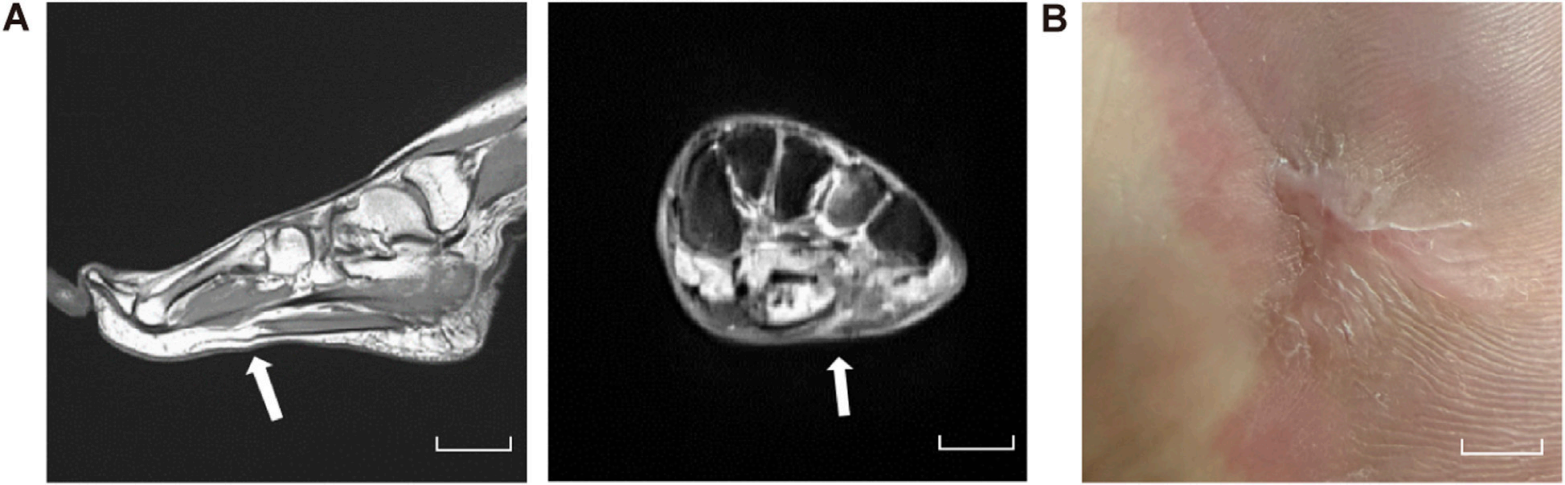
At the 1-year follow-up. (A) MRI of the left plantar foot. Scale bars, 5 cm. (B) Latest figure of wound healing. Scale bars, 1 cm.
